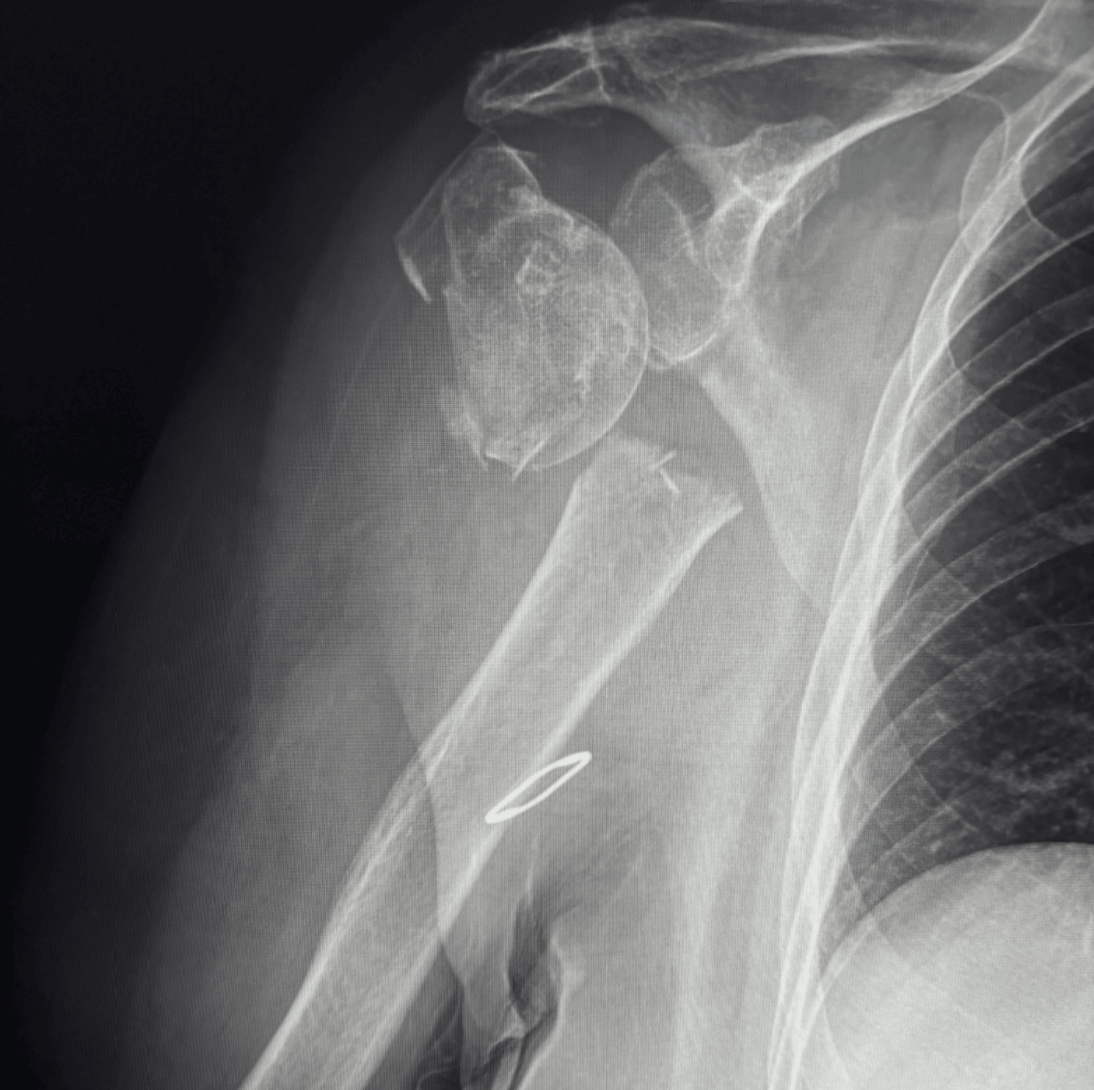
Radiograph of a proximal humerus fracture
